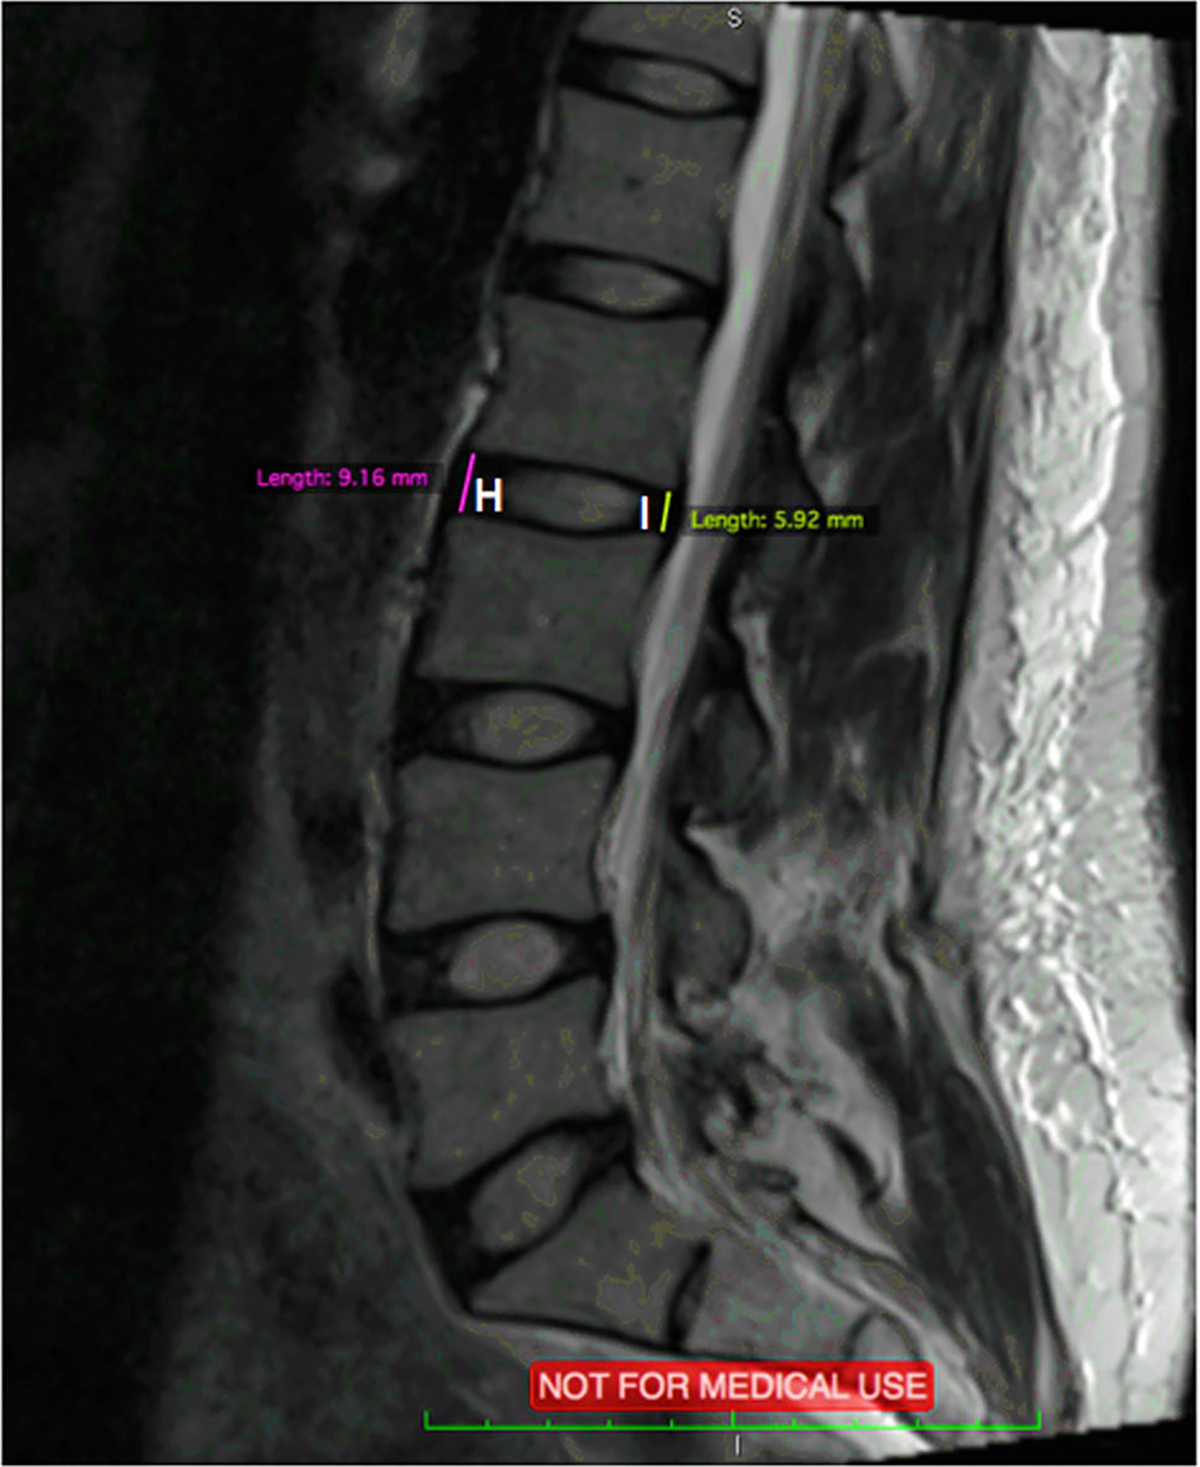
Sagittal MRI scan with measurements of H and I
